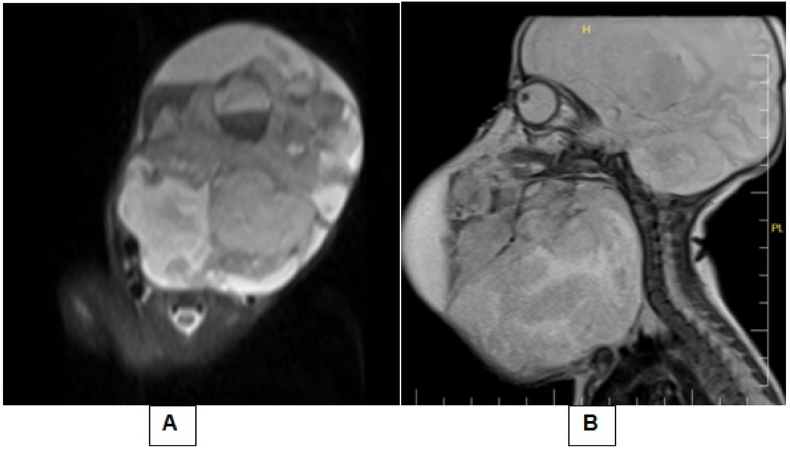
Axial T2 (A) and Sagital T1 (B) pre-contrast MRI scans show a massive well-defined multi-loculated complicated neck mass with considerable mass impact. The solid component has intermediate T2 and T1 signal intensities, whereas the cystic component exhibits high T2 and low T1 signal intensities, The esophagus and airway have been shifted.
